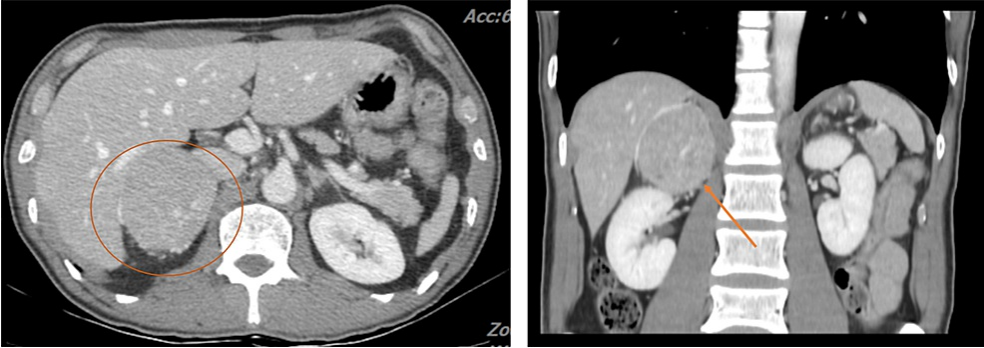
CT scan shows right adrenal mass (Left: circle, Right: arrowhead)
